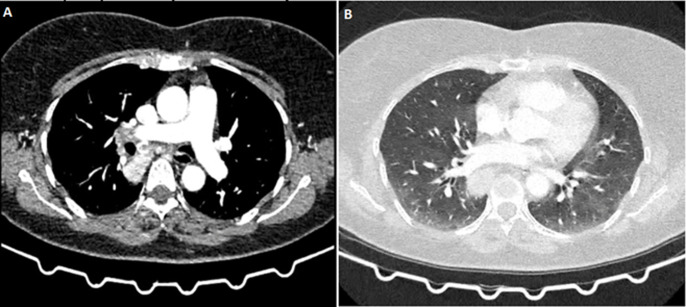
chest CT imaging of a posterior mediastinal mass: A) mediastinal window, highlighting the mass, while panel; B) parenchymal window for better visualization of the surrounding lung tissue
